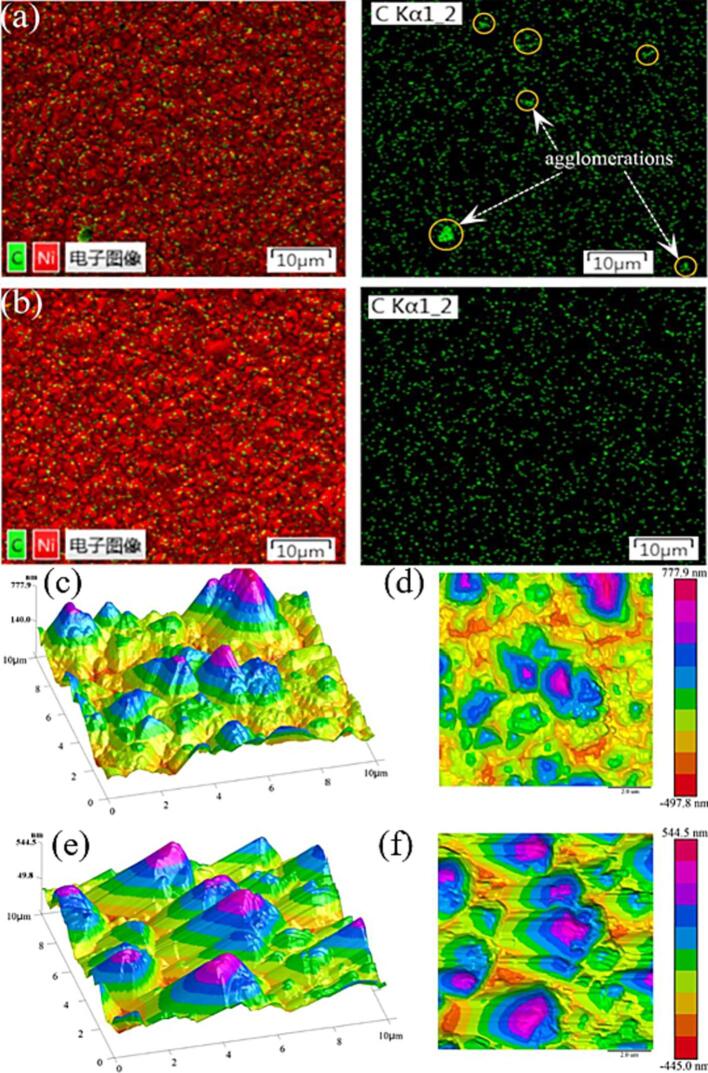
Electrodeposition of Ni/diamond coatings (a) elemental distribution of electrodeposited coating under magnetic stirring and (b) ultrasonic agitation, surface topography of electrodeposited coating under magnetic stirring (c,d) and ultrasonic agitation (e,f). Adjusted from reference [31] with permission of Elsevier.
